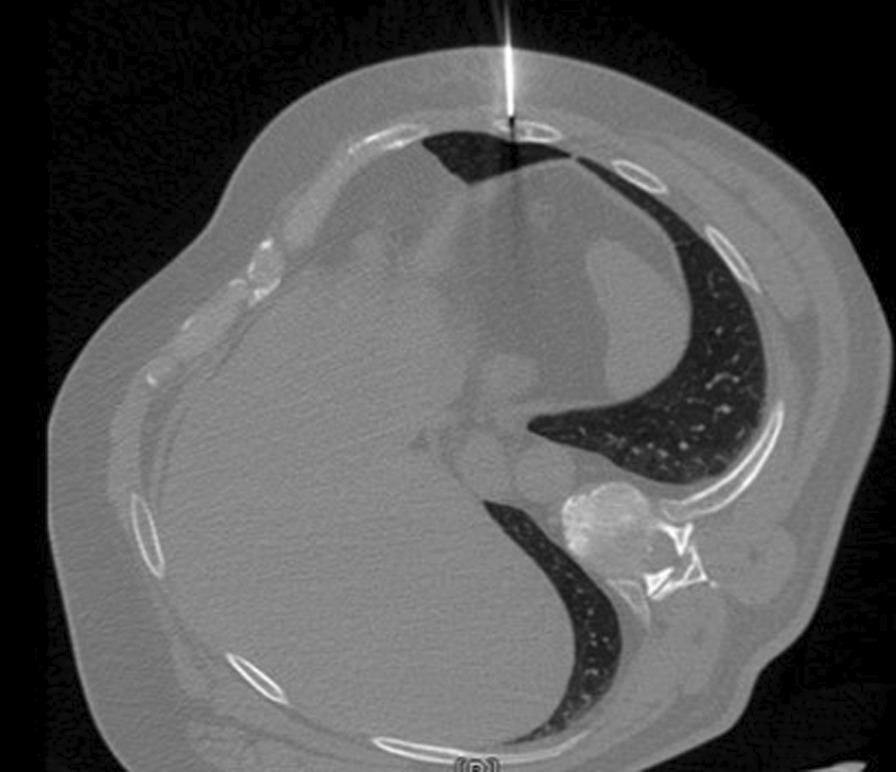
Percutaneous marker. Positioning of the percutaneous marker on the lesion under CT-guidance
